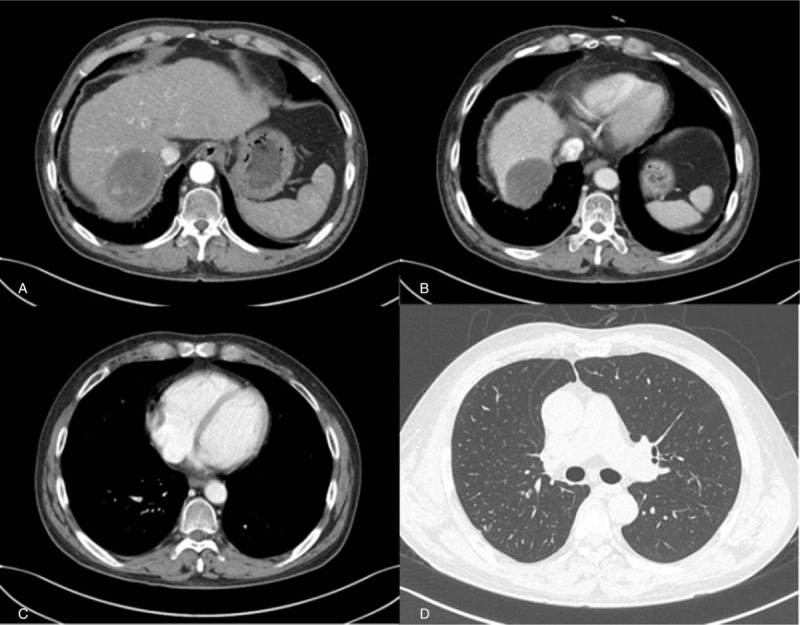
Two years later, follow-up abdominal computed tomography (CT) revealed a 6.4 × 5.2-cm-sized and significantly reduced necrotic hepatocellular carcinoma in the right hepatic lobe, without tumoral enhancement (A). Nearly resolved tumoral thrombosis in the suprahepatic inferior vena cava (B) and complete resolution of the right atrium metastasis was seen (C). No detectable pulmonary nodules in both lungs on Chest CT (D).
